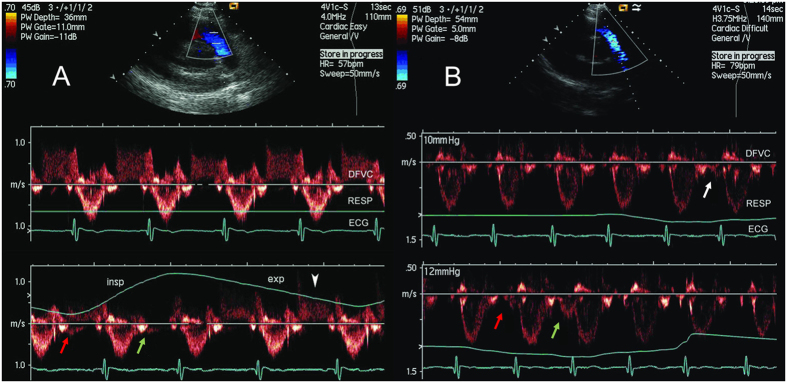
(A) Effects of respiration on the pulmonary regurgitation jet velocity and the pulmonary valve diastolic opening. The top panel shows a 2D echocardiogram in the parasternal short-axis view at the aortic valve level from a subject in the pulmonary regurgitation (PR) method group. The middle panel depicts the Doppler flow velocity curve (DFVC) of this healthy subject with mild PR. The Doppler flow velocity curve of the middle panel was recorded at end-expiration during breath holding. The peak PR velocity was slightly less than 1 m/sec, indicating that the pressure difference across pulmonary valve (PVPG) is close to 4 mmHg based on the modified Bernoulli equation. Note that the respiration curve is flat during the breath hold (RESP). The bottom panel showed that with the onset of inspiration (insp), the PR velocity decreased sharply and became erratic at the pulmonary valve orifice (red arrow, flow signals above and below the baseline). Subsequently, at end-inspiration, pulmonary arterial pressure was equal to or less than that in the right ventricle, and a discernable forward pulmonary arterial flow was detected (green arrow, PR nearly absent), indicating natural pulmonary valve diastolic opening. The PR velocity gradually increased with expiration (exp) and the waveform nearly normalized by end-expiration (arrowhead). (B) Exaggerated inspiratory effort leads to erroneous measurement of PVPG during the Muller maneuver. The top panel shows the same view as that in A, but from a subject in the Catheterization group. The middle panel shows the DFVC with the onset of a premature flow signal (arrow) at end-diastole during gradual Muller maneuver, indicating that at 10 mmHg of negative pressure, the pressure in the pulmonary artery is just below that of the right ventricle, the pulmonary valve opens prematurely, and forward flow occurs. The bottom panel demonstrates that with an exaggerated Muller maneuver, the premature flow was more prominent and was of greater velocity (green arrow) at 12 mmHg of negative pressure, resulting in an overestimation of PVPG.
